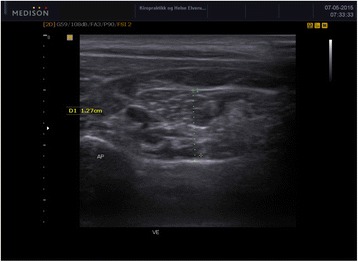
Ultrasound image of the Dce in transverse view at C5. The caliper is placed 90° to the lamina of C5 where the rater considered the muscle to be at its thickest and up to the echogenic line of the hyperechoic fascia between the Sscerv and Sscap
